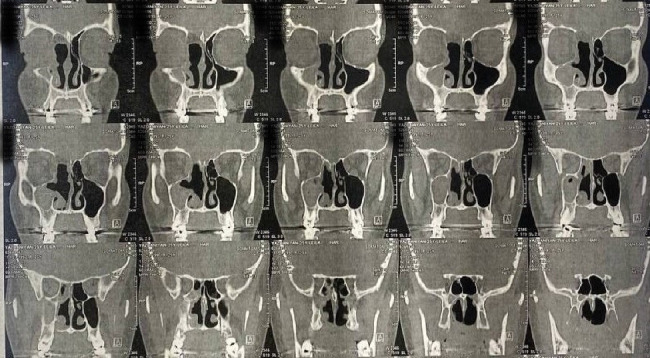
The patient's postoperative CT scan after a year.
